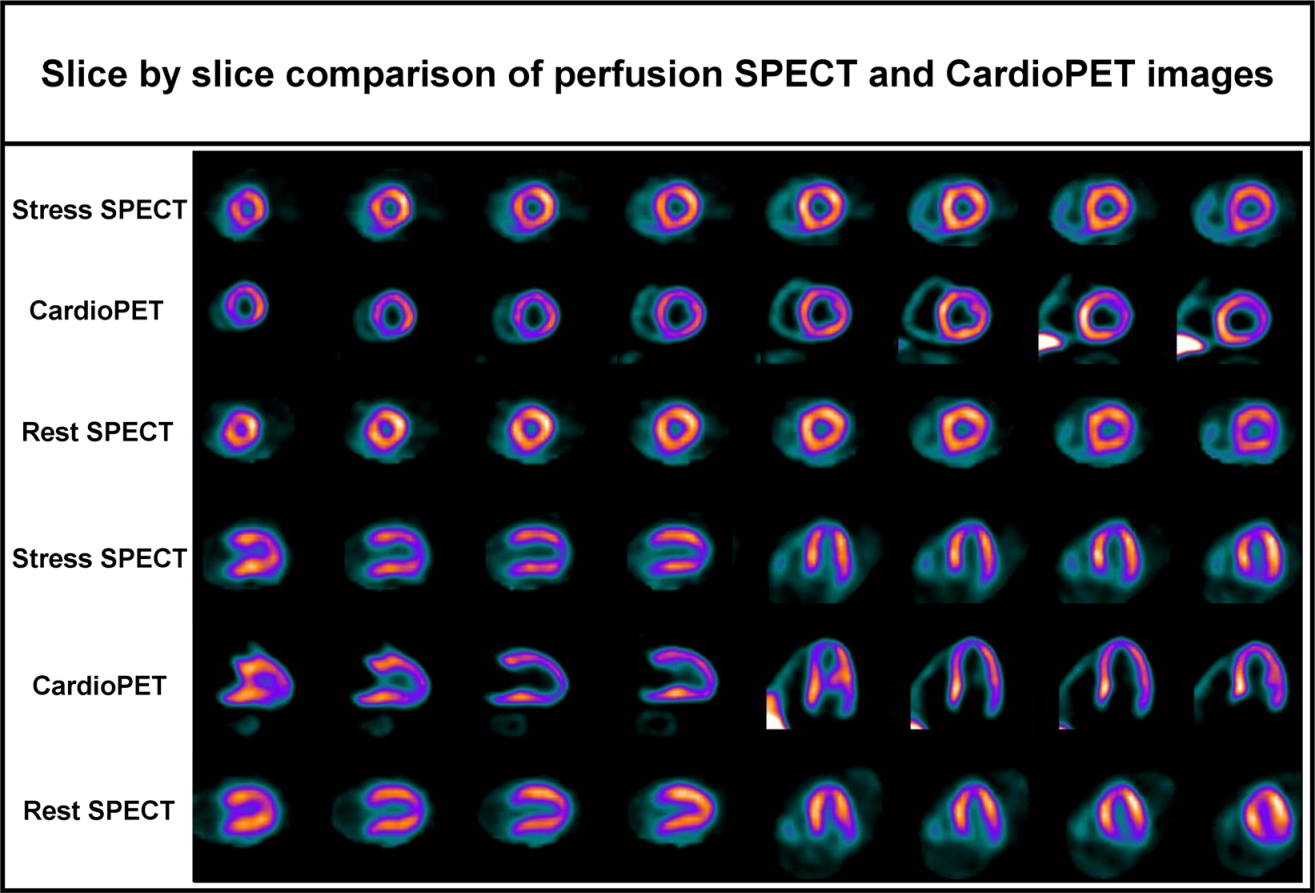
Slice by slice comparison of the stress perfusion SPECT, CardioPET, and rest perfusion SPECT images allowing to appreciate the more extensive defects on the cardioPET scan in relation to the Tc-99m-Sestamibi stress perfusion SPECT scan
